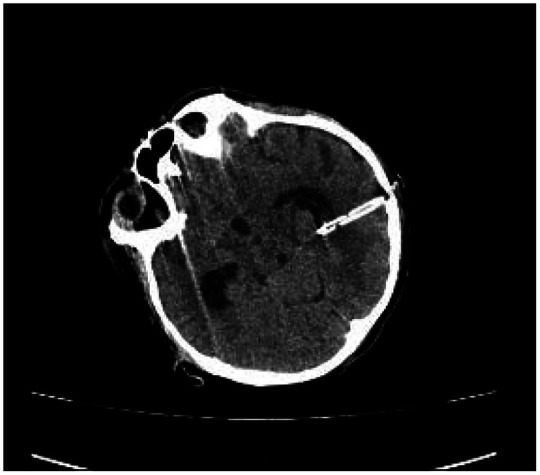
CT reveals an external ventricular drainage catheter positioned in the posterior horn of the left lateral ventricle.
